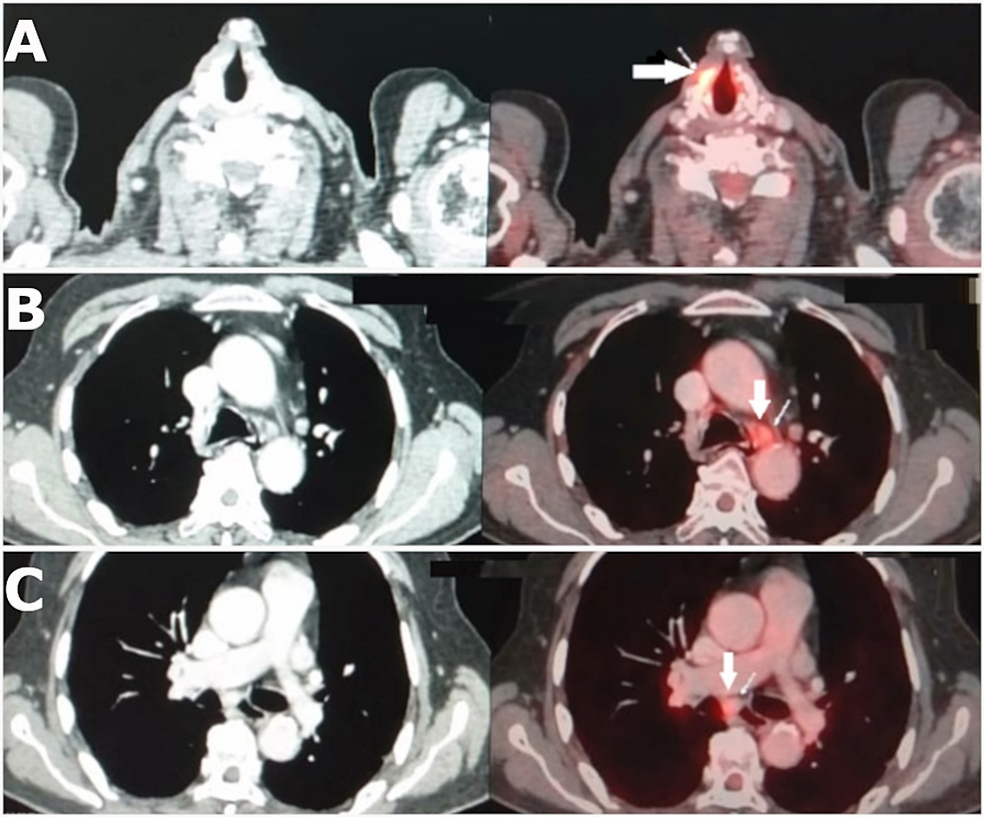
PET-CT for the spread of the carcinoma of the larynx (A) Transaxial CT and fused PET-CT images showing focal FDG avidity along anterior half of right vocal cord (arrow); (B) transaxial CT and fused PET-CT images showing mild degree FDG avid left lower paratracheal node (arrow), which appeared nonspecific reactive in nature; (C) transaxial CT and fused PET-CT images showing mild degree FDG avid subcarinal node (arrow), which appeared nonspecific reactive in nature. FDG: fluorodeoxyglucose, PET-CT: positron emission tomography and computed tomography.
